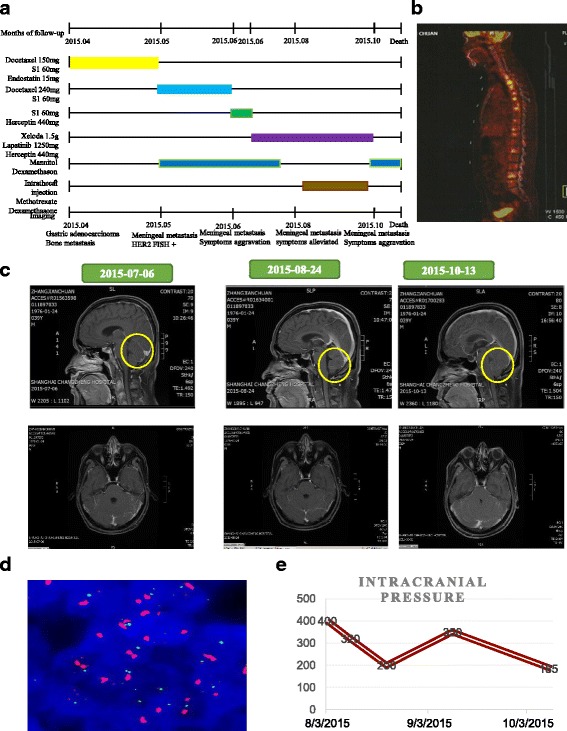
The course of treatment. a Timeline describing clinical course, treatments administered and selected imaging assessments. b Positron emission tomography-computed tomography (PET/CT) scan demonstrated widely metastatic disease throughout his skeleton. c MRI of the brain showed edema of cerebellum at indicated time point. d HER2 testing of these tumor cells by fluorescent in-situ hybridization (FISH). e, The changes of intracranial pressure during the treatment process
